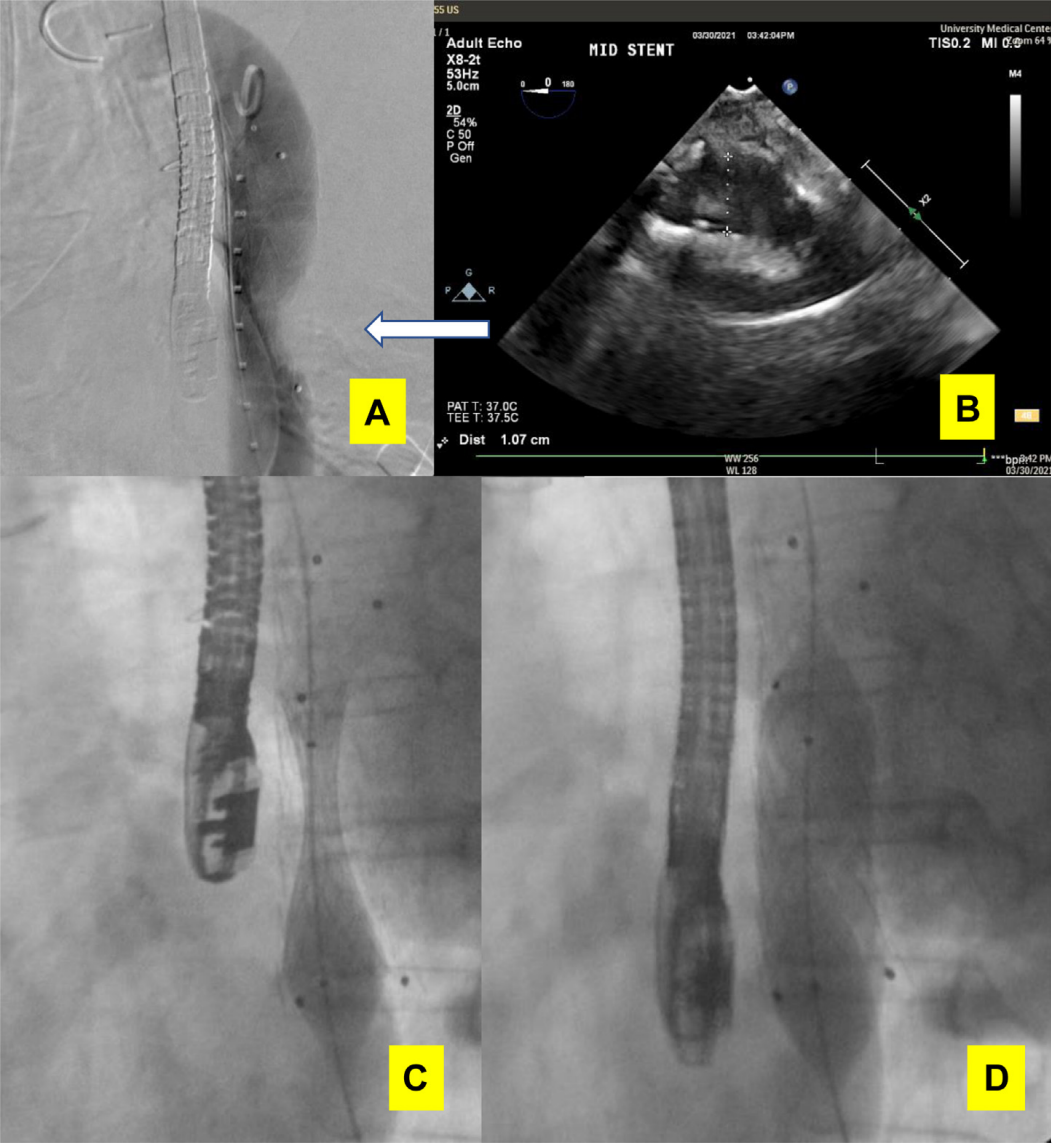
Intraoperative simultaneous fluoroscopy and live transesophageal echocardiography (TEE) monitoring during Endurant-II stent-graft (Medtronic) and Palmaz stent (Cordis) placement.
